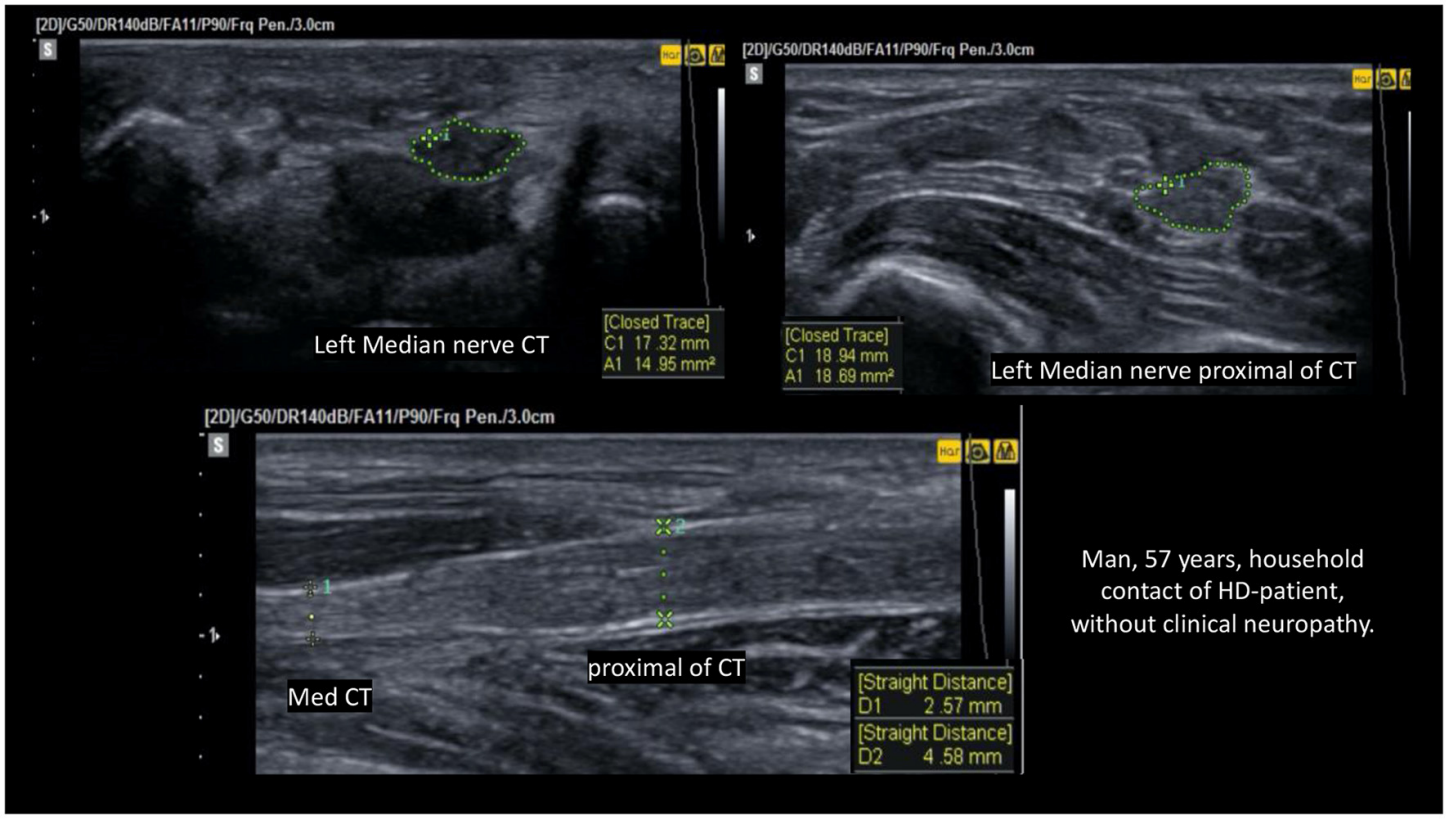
Fusiform thickening of the left median nerve regarding focality (ΔTpT) CSA pCT > CSA CT; ΔTpT = 18.69 −14.95 = 3.7 mm^2^ > ULV (upper limit value).
